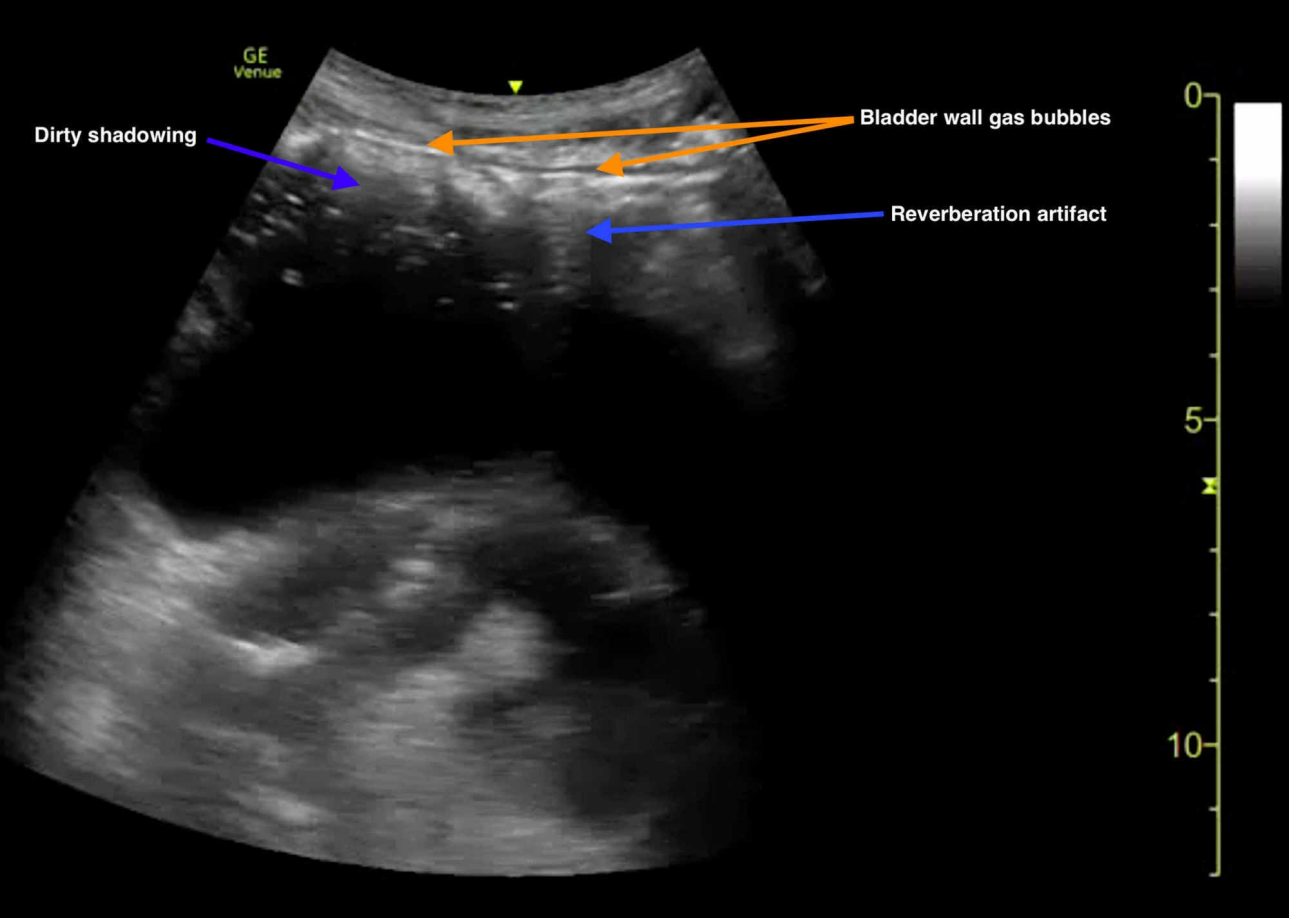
Point-of-care ultrasound demonstrating an air-fluid level of the bladder. Gas bubble of anterior bladder wall is indicated by multiple hyperechoic foci (orange arrows) with corresponding reverberation artifact and dirty shadowing (blue arrows).
